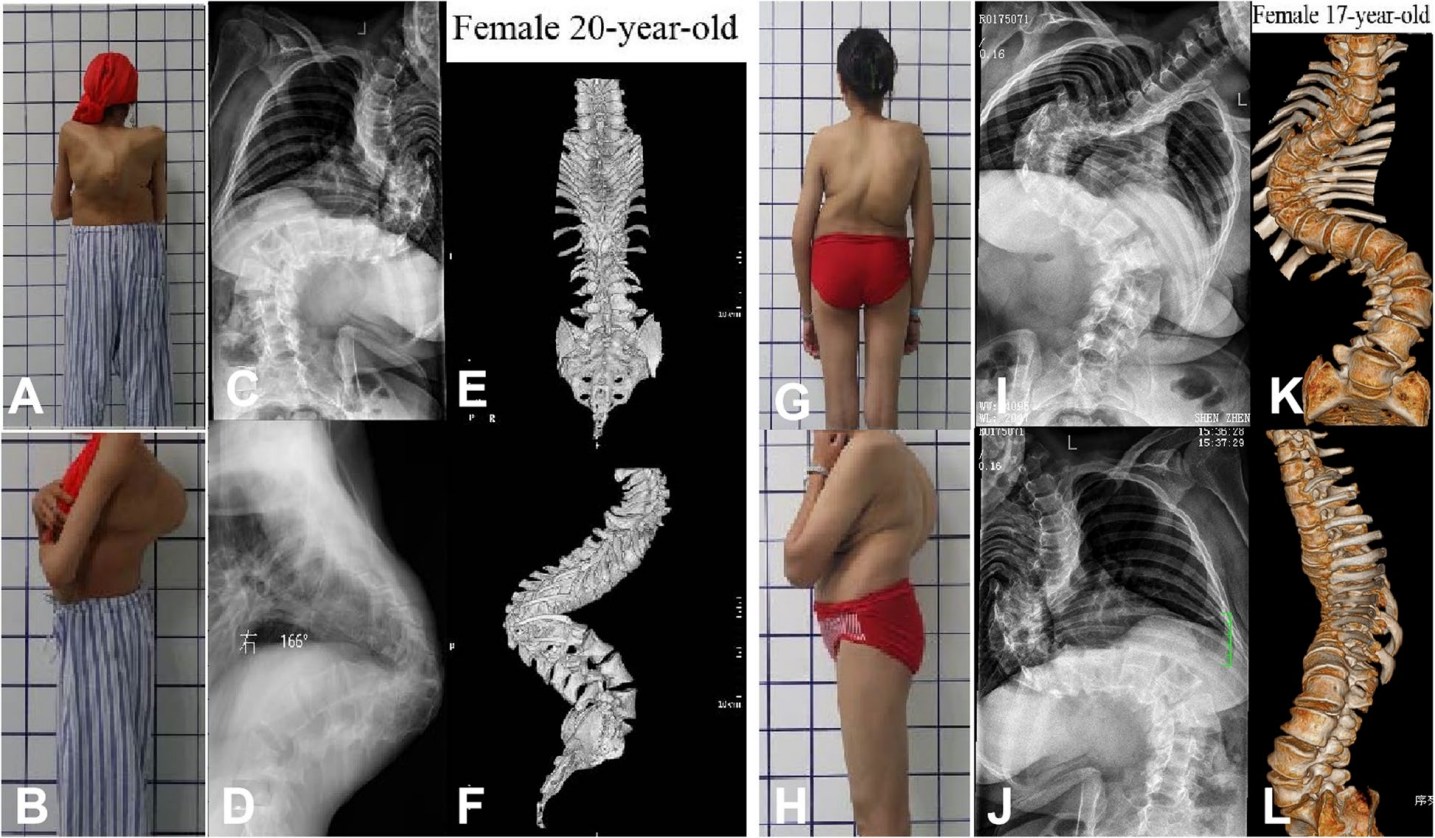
Female, 22 years old, severe angular kyphosis. A, B, C, D, E and F Preoperative diagnosis was kyphosis: Cobb angle 166° by profile, X-ray after bending, three-dimensional CT. Female, 17 years old, G, H, I, J, K and L) Preoperative diagnosis was kyphosis: Cobb 99° and scoliosis Cobb 97° by profile, X-ray after bending, three-dimensional CT
